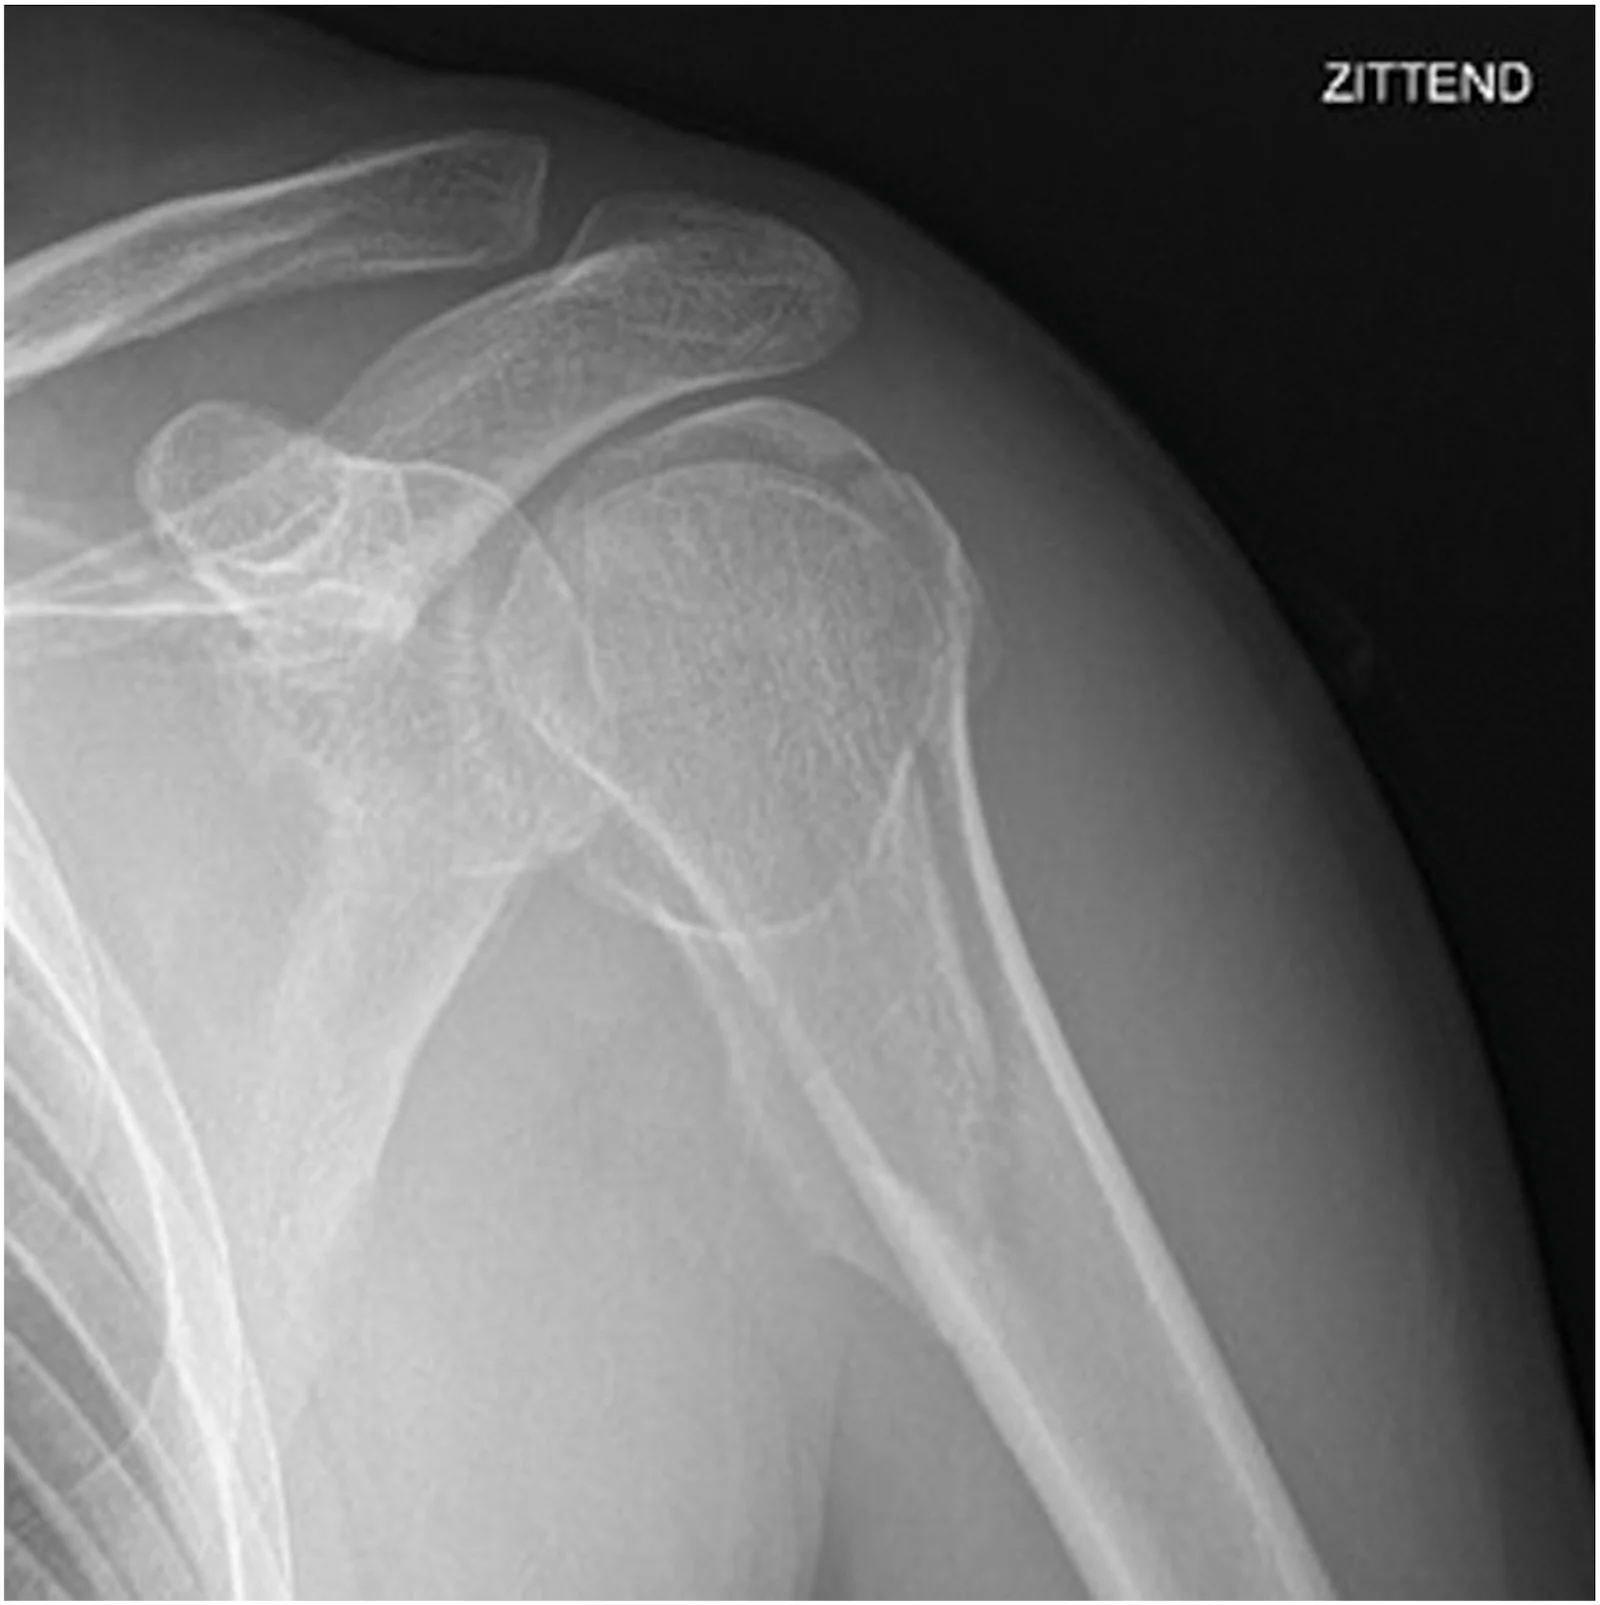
Preoperative conventional anteroposterior radiograph of the left shoulder showing a three-part proximal humeral fracture with a light bulb sign.
